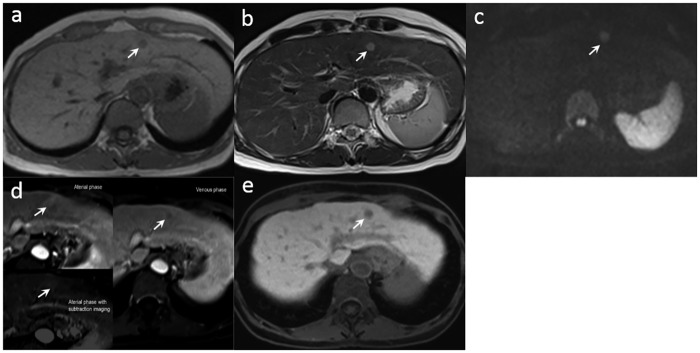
A 63-year-old male with a well-differentiated HCC (arrow) at S3 of the liver underwent gadoxetic acid-enhanced MR imaging and hepatectomy. (a) The tumor was hypointense on the T1-weighted image. (b) The tumor depicted hyperintensity on the T2-weighted image. (c) The tumor depicted hyperintensity on the diffusion-weighted image. (d)The tumor was isointense to the adjacent liver parenchyma in the arterial phase, but was hypointense in the venous phase of the dynamic MRI study. (e) The tumor was hypointense on the Gd-EOB-DTPA-enhanced hepatobiliary phase T1-weighted image.
